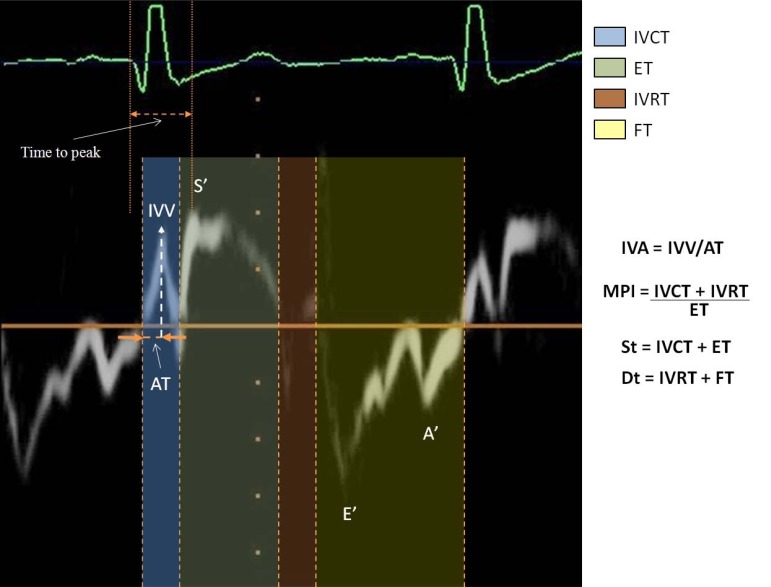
Spectrogram of PW-TDI. In particular: Tissue Doppler – derived myocardial systolic and diastolic velocities, and time intervals.
